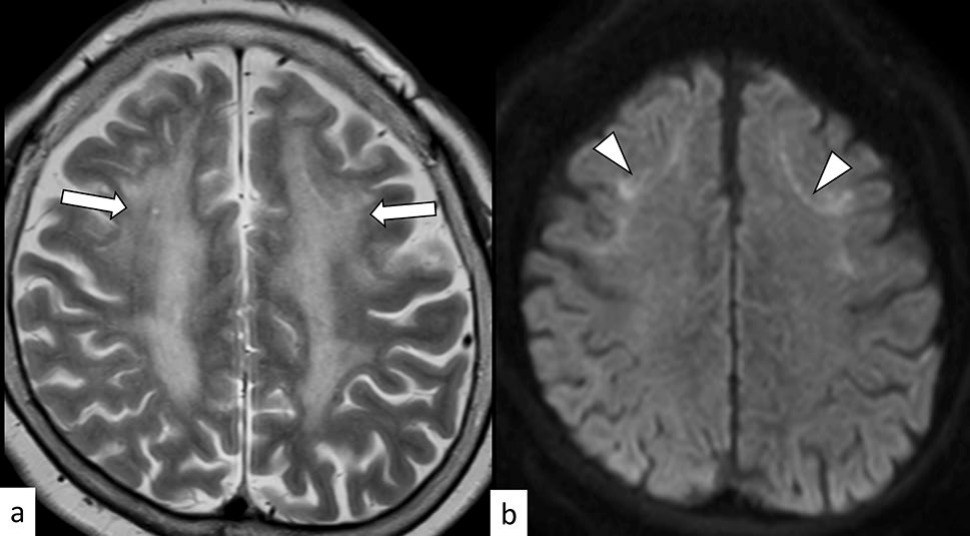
A 59-year-old woman with NIID. T2-weighted axial image shows hyperintensity in the white matter with atrophy (a, arrows). DWI shows hyperintensity bands along the corticomedullary junction (b, arrowheads). The paravermal FLAIR hyperintensity, which can be frequently observed in NIID, is not present in this case (not shown)
